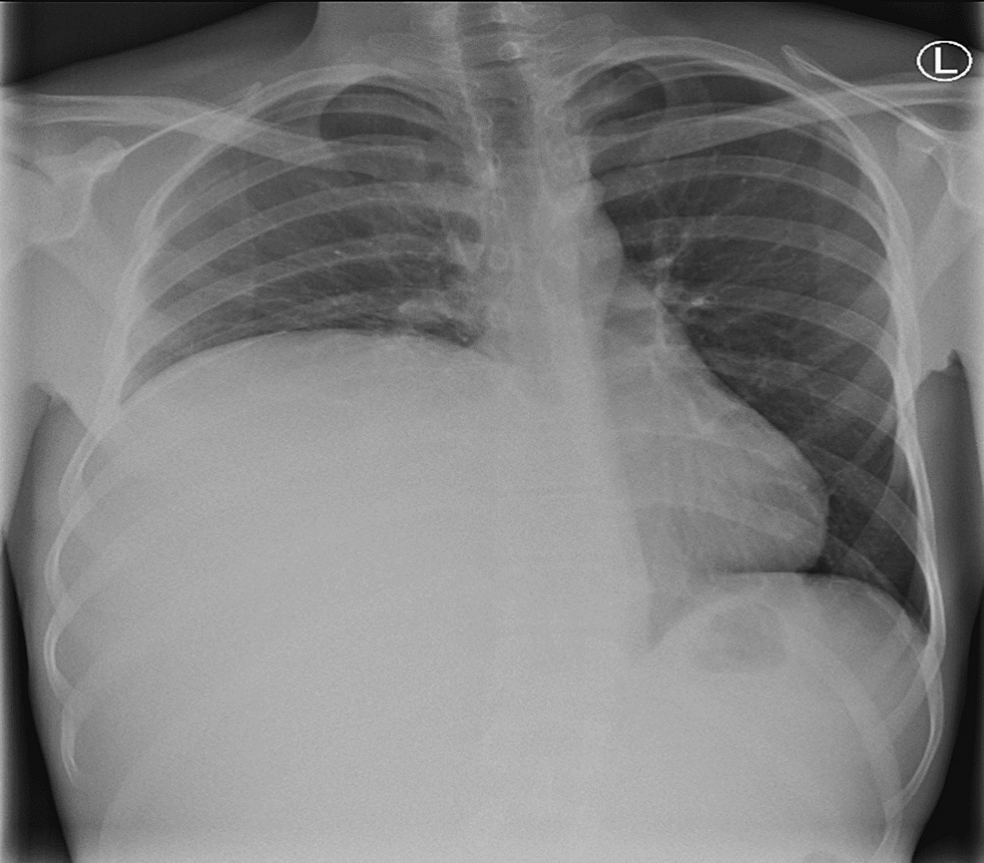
Chest x-ray demonstrates a large liver lesion occupying most of the right side of the chest cavity and displacing mediastinal structures to the left.
